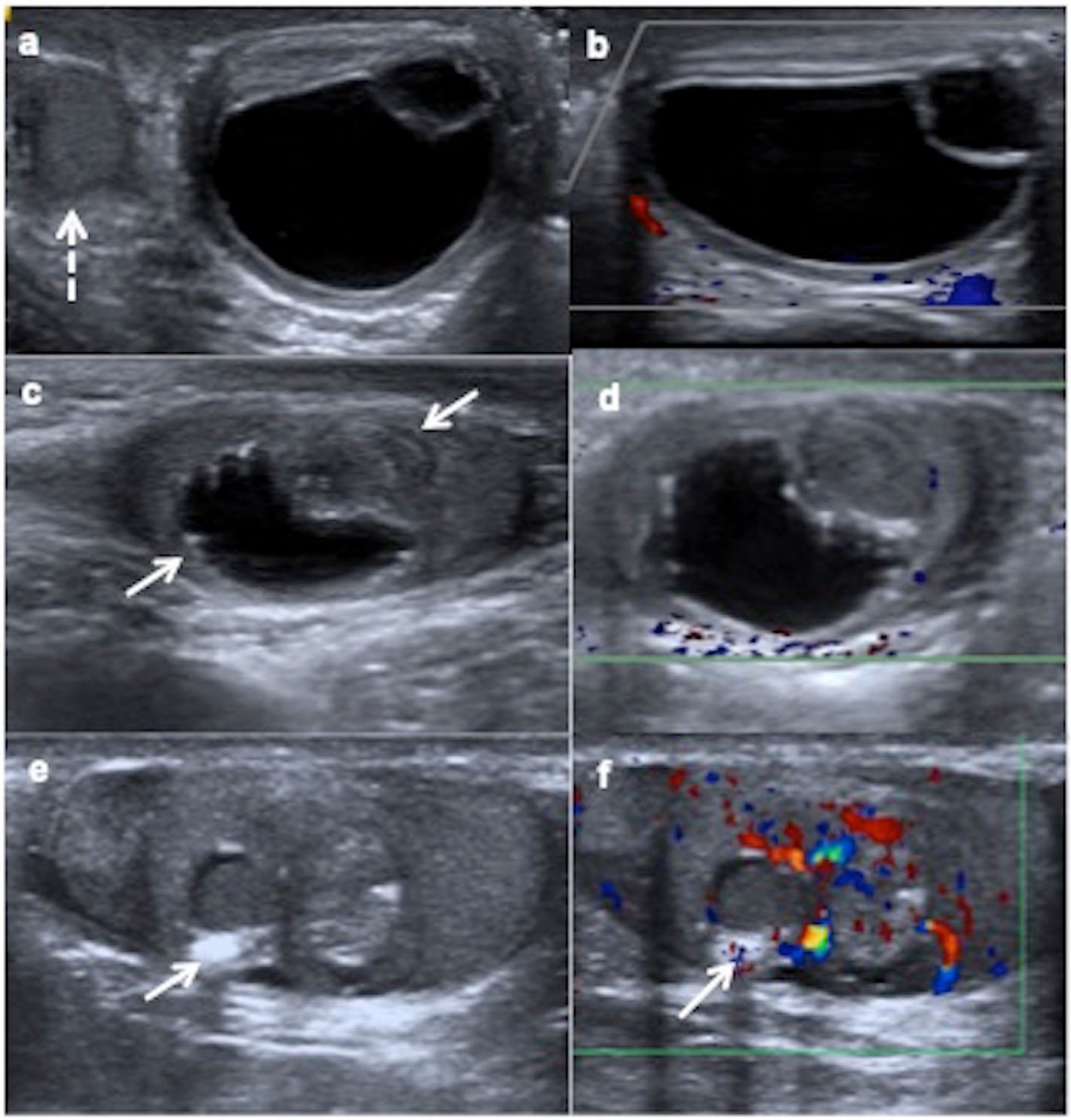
Mature teratomas, prepubertal-type: ultrasound appearances. a, b Ultrasound and colour Doppler of a 10-month-old boy with a swollen and tender left testis show an avascular cystic mass completely replacing the testicular parenchyma, an orchiectomy being required. Right testis (discontinuous arrow). c, d Ultrasound and colour Doppler of a 8-month-old boy with a painless scrotal left mass show a mixed solid-cystic mass (arrows) without blood flow in the solid part of the lesion. Orchiectomy is practised because of the big size of the lesion. e, f Ultrasound and colour Doppler of a 8-year-old boy with a swollen and hard right testis show a heterogeneous solid mass, calcifications (arrow) and blood flow inside. A testis sparing surgery is practised since sufficient salvage parenchyma is visible
